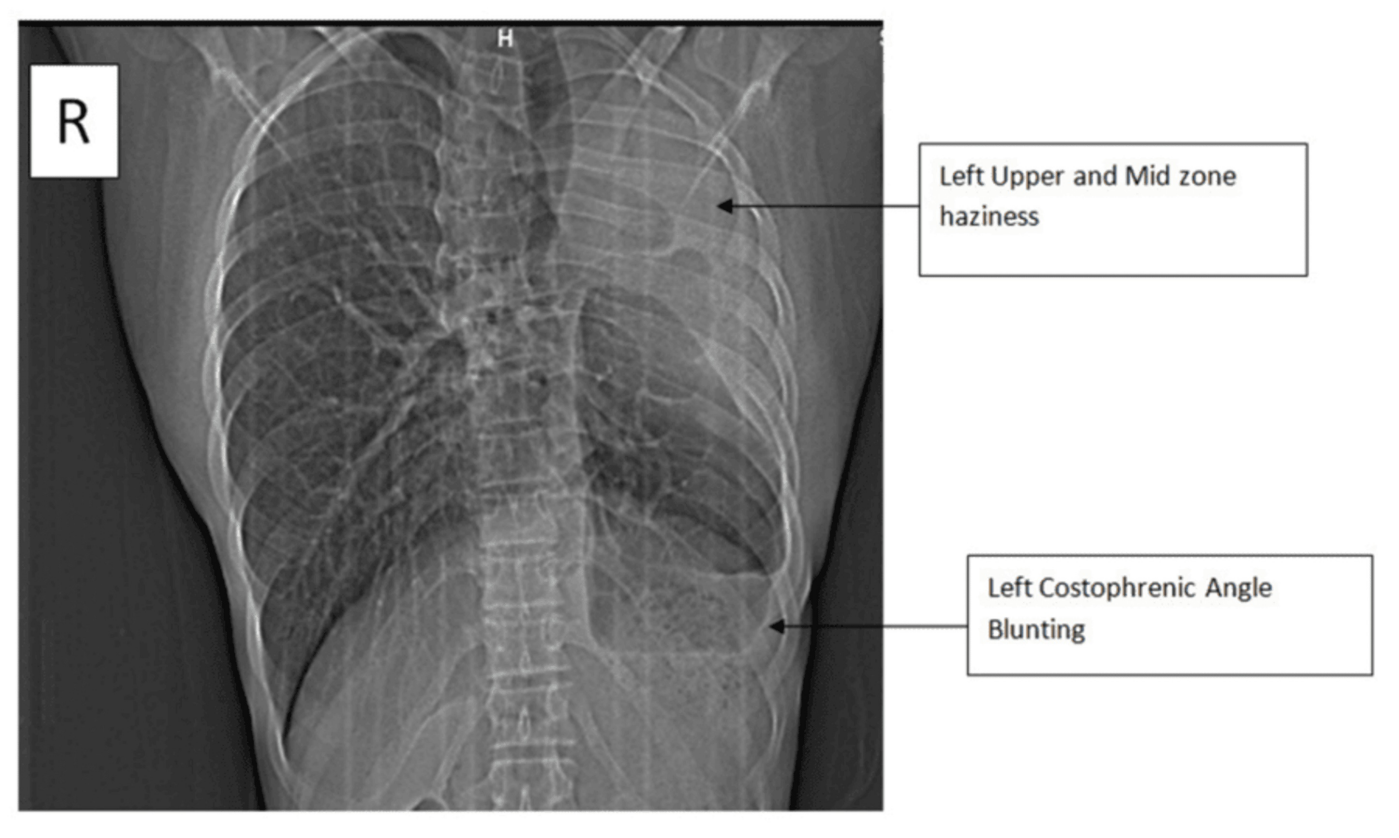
Chest X-ray posteroanterior view The image shows left upper and mid zone haziness and left costophrenic angle blunting (black arrows)
